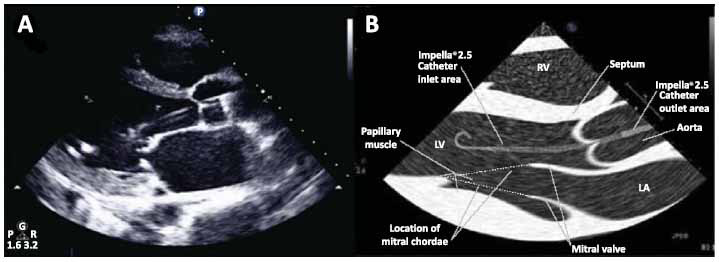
Parasternal long-axis view of Impella positioned in the left ventricle (adapted from Burzotta *et al.*, no changes were made to the original image) [20] (https://creativecommons.org/licenses/by-nc-nd/4.0/).
